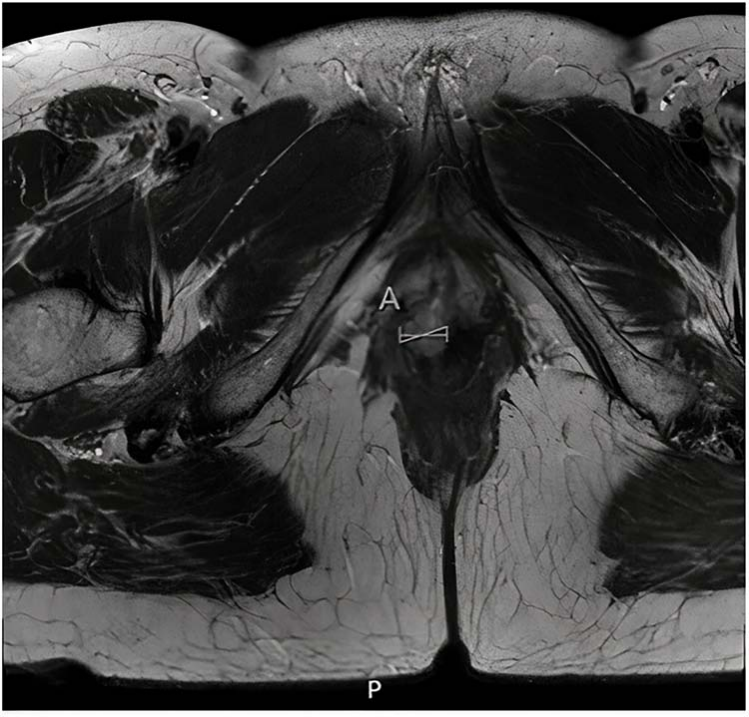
Axial view of diagnostic MRI-rectum with the solid tumour indicated with the linear mark ‘A’.
